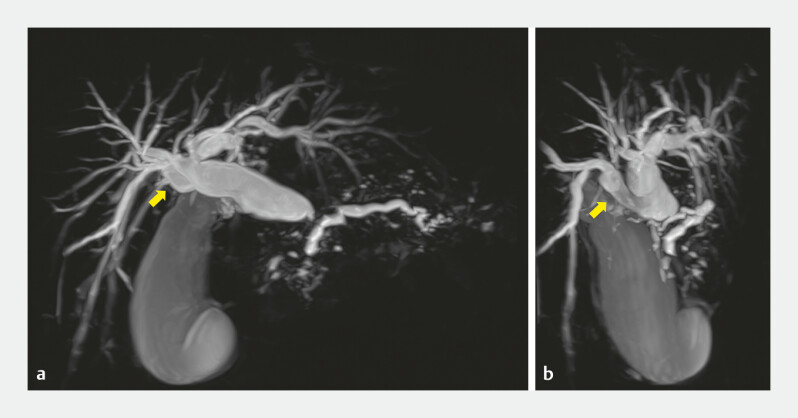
Preoperative magnetic resonance imaging showed an infraportal anomaly of the right posterior bile duct (arrow).
